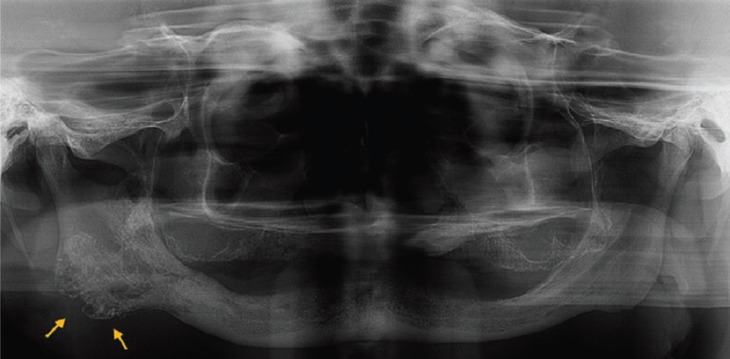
Panoramic radiography showing a multilocular osteolytic radiolucency at the right mandibular angle (arrows), with irregular and indefinite margins, extending to ascendant ramus.
